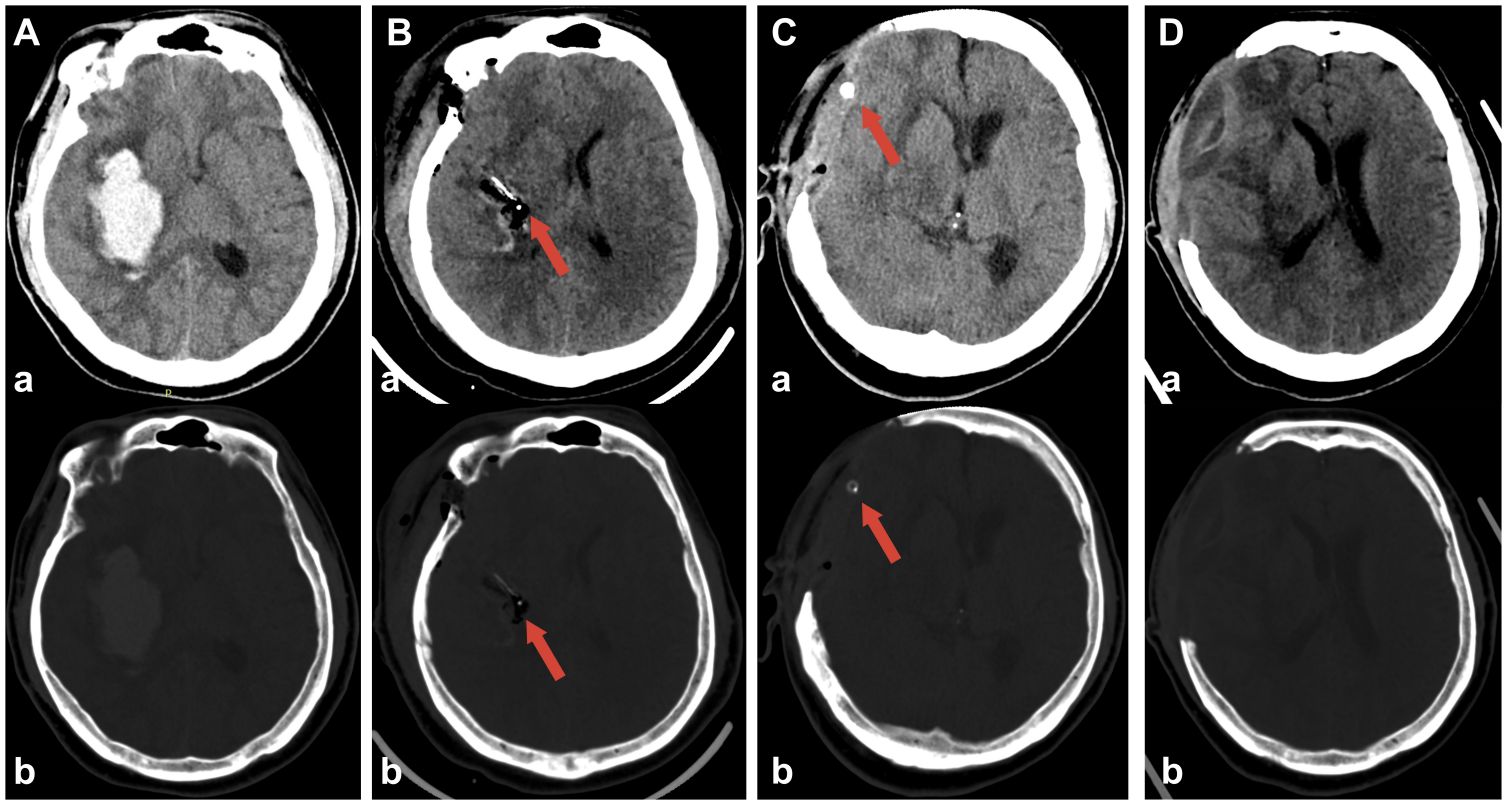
Representative case demonstrating dynamic changes in a patient treated with RA-MIS. (A) Preoperative CT scan showing intracerebral hemorrhage in the left basal ganglia. (B) Four hours after robot-assisted minimally invasive surgery, the CT scan confirms successful placement of the drainage catheter into the hematoma cavity. (C) Following urokinase injection into the hematoma cavity, accumulated blood was successfully evacuated; the CT scan at 24 h post-operation shows a significant reduction in the hematoma size. (D) CT scan at 3 days post-operation demonstrating near-complete resolution of the hematoma. Red arrows indicate the placement of the drainage catheter in each scan, with ‘a' for non-contrast images and ‘b' for bone window.
